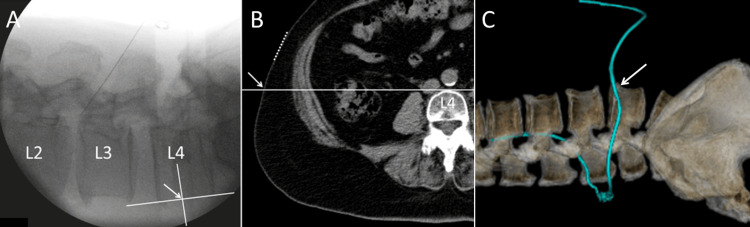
Lateral Abdominal Approach at the Skin Incision Site (A) Fluoroscopic View; (B) Abdominal Computed Tomography; (C) Three-Dimensional Computed Tomography The patient is positioned in the left lateral decubitus position, and a 4 cm skin incision (dotted line) is made 3 cm anterior to the L4 vertebral body level (arrow), guided by fluoroscopy.
